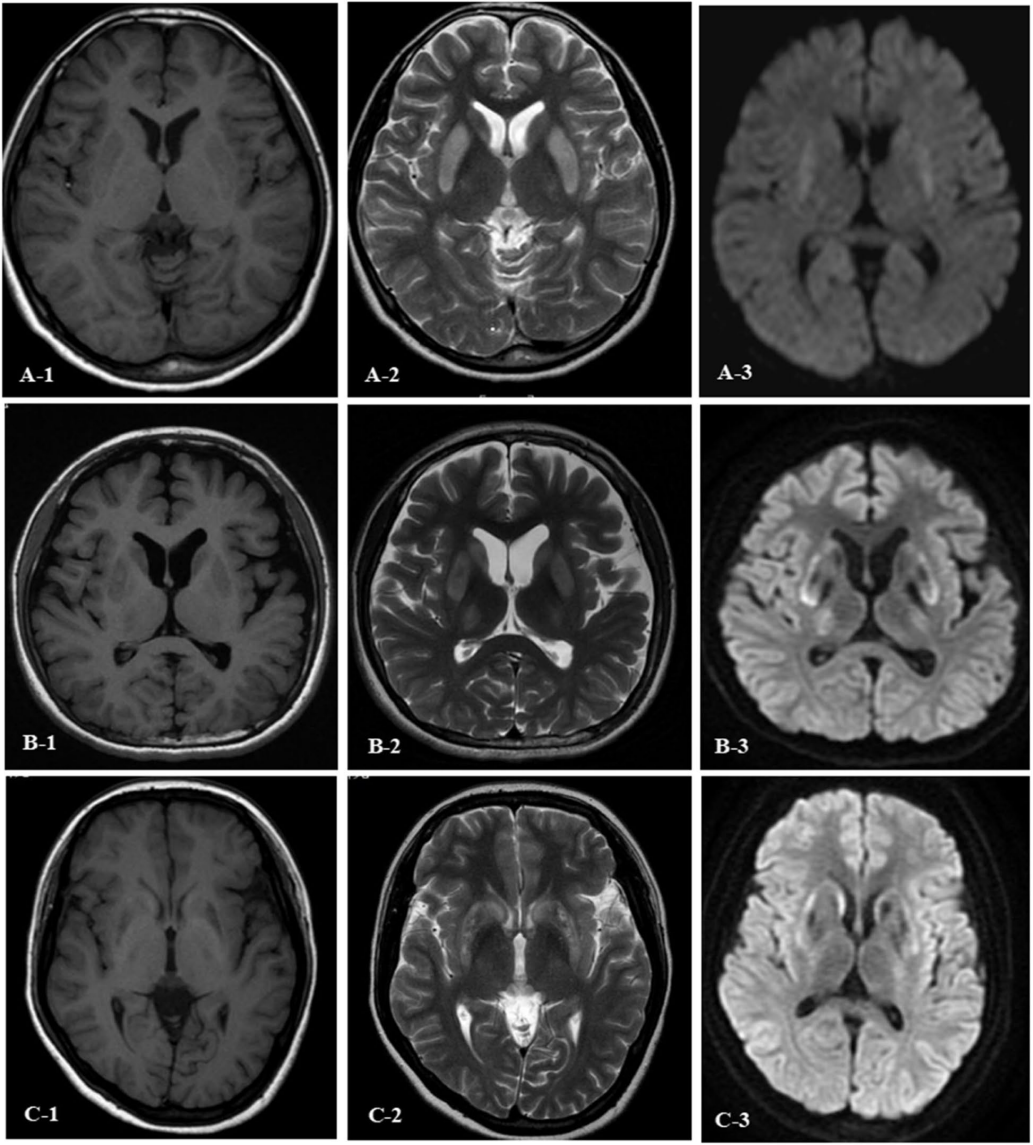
Magnetic resonance imaging (MRI) of the brain in 3 patients with purely hepatic Wilson disease (WD) after appearance of neurological symptoms. A1-A3 images were from a 14-year-old female patient with purely hepatic WD at 3 weeks after appearance of neurological symptoms with the high signal intensity in bilateral putamen and caudate nucleus on T2-weighted imaging (T2WI) and diffusion-weighted imaging (DWI), and the signal intensity was low at the corresponding position on T1-weighted imaging (T1WI). B1-B3 images were from an 18-year-old female patient with purely hepatic WD at 4 weeks after appearance of neurological symptoms, with the high signal intensity (local low signal intensity) in the bilateral putamen, thalamus, and caudate nucleus on T2WI and DWI-MRI, and the signal intensity was low at the corresponding position on T1WI. C1-C3 images were from a 24-year-old female patient with purely hepatic WD at 6 weeks after appearance of neurological symptoms, with the high signal intensity (local mixed signal intensity) in the bilateral putamen and caudate nucleus on T2WI and DWI-MRI, and the low signal intensity at the corresponding position on T1WI
